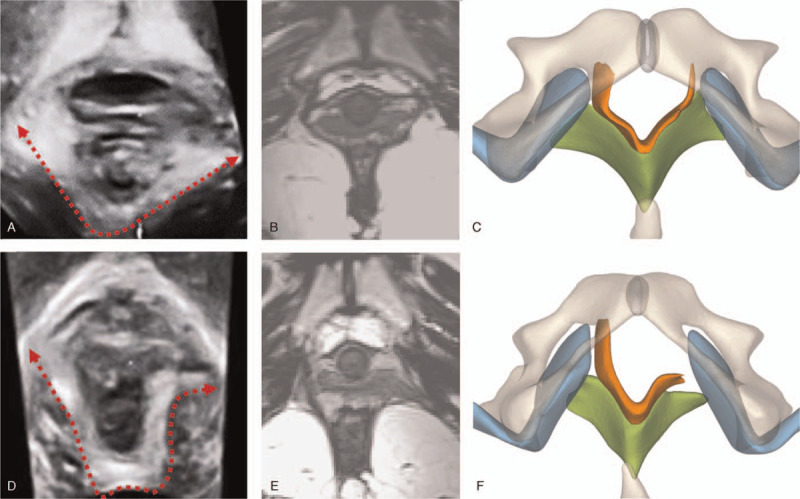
The ultrasound images, magnetic resonance images and three-dimensional models of the two cases are shown (A-F). Each line represents the same case respectively. Images in the first column are ultrasound images, images in the second column are magnetic resonance images, and images in the third column are three-dimensional pelvic models. Images in the first line (A-C) show the left puborectalis tear (a complete tear on 3D-US, a partial tear on MRI). The images in the second line (D-F) show the left puborectalis tear with iliococcygeus tears (a complete tear on 3D-US and MRI). Red dotted lines show the morphology of the puborectalis (A, D). Showing muscles and pelvic bone in color, the puborectalis in orange, the iliococcygeus in green, the internal obturator muscle in blue.
